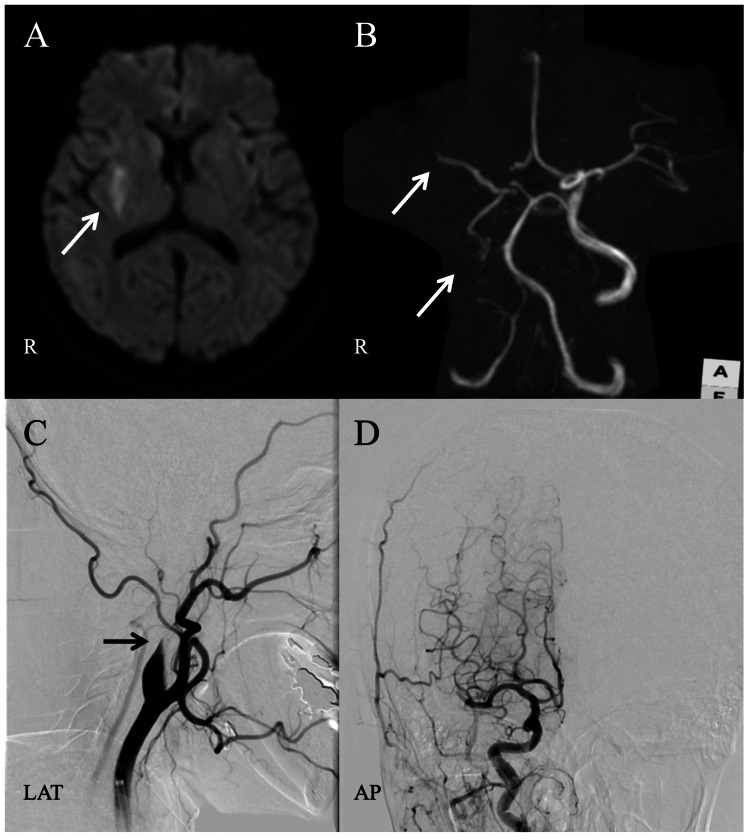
Cerebral imaging findings in this case. (A) Brain magnetic resonance imaging findings. The diffusion-weighted image on admission shows an infarct in the right hemisphere. (B) Magnetic resonance angiography on admission shows a right internal carotid artery occlusion. (C) The right carotid artery angiogram shows an occlusion at the cervical internal carotid artery. (D) After mechanical thrombectomy, the right internal carotid artery was partially recanalized. LAT, lateral view; AP, anterior-posterior view
